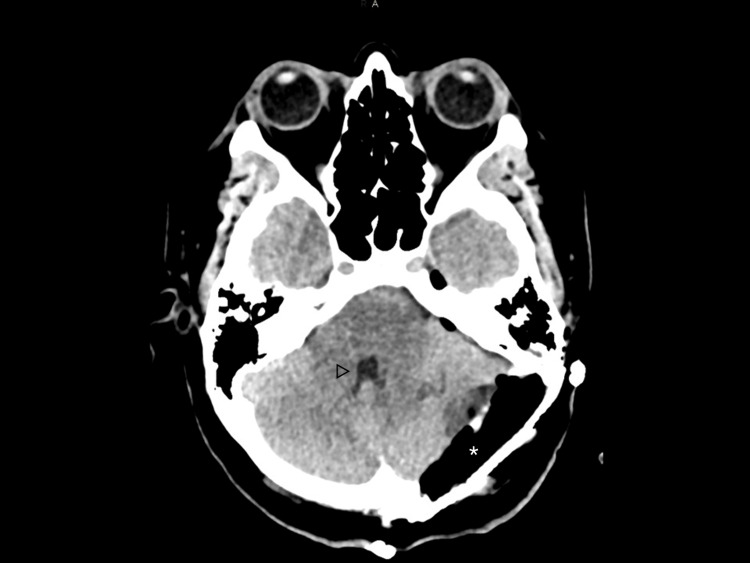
Postoperative CT showing resection of the lesion. Fat graft (asterisk) in the epidural space and opening of the fourth ventricle (triangle).
